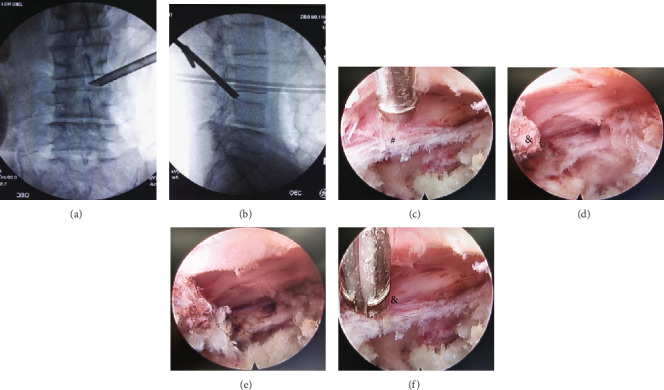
Radiographs showing that the working cannula has been successfully inserted into the surgical area at the L3-4 level: (a) anteroposterior radiographs and (b) lateral radiographs. Endoscopic view of the surgical procedure: endoscopic views of (c) before and (d) after removal of the ligamentum flavum and the extruded disc. Endoscopic views of (e) before and (f) after removal of the hyperplastic facet joint to relieve compression from the lateral recess.
